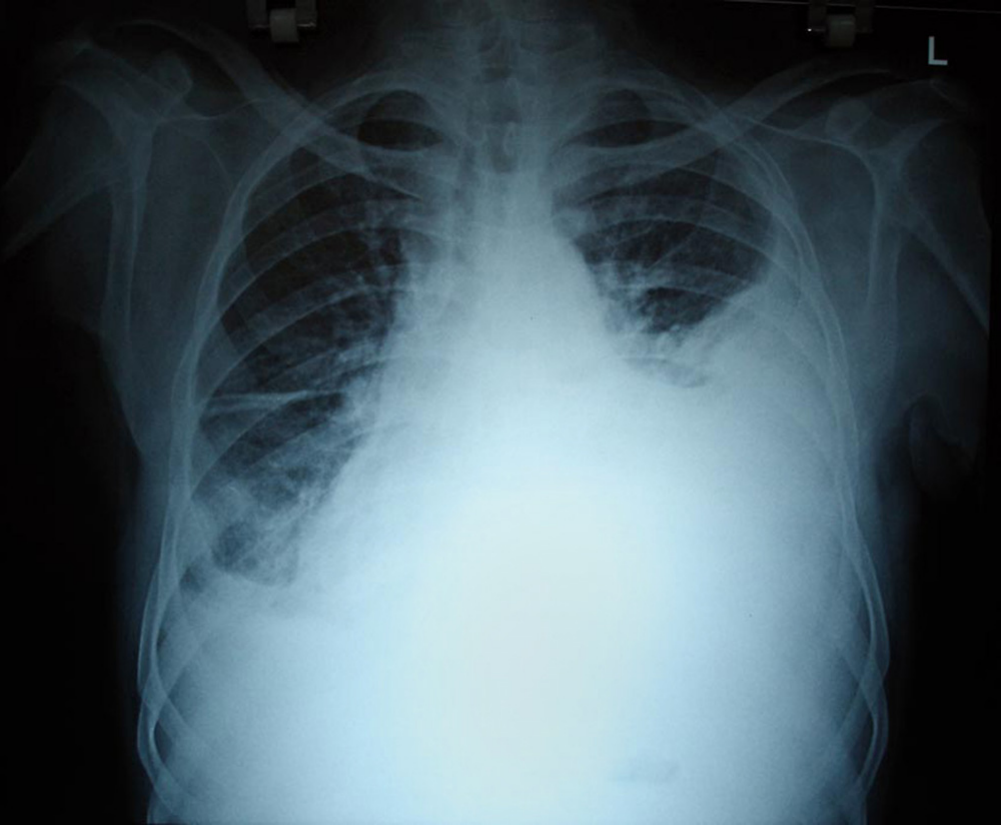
X-ray chest on admission. X-ray of the chest (PA-view), showing a left sided massive pleural effusion. This X-ray was taken on admission.
